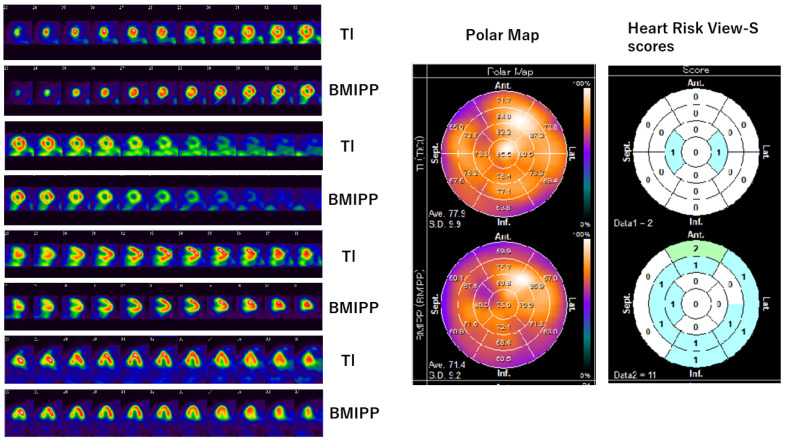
Tl/BMIPP dual-isotope myocardial scintigraphy of a patient. (Left) SPECT images are arranged in order from top to bottom: left ventricular short-axis planes of ^201^Tl and ^123^I-BMIPP, long-axis planes of ^201^Tl and ^123^I-BMIPP, and horizontal planes of ^201^Tl and ^123^I-BMIPP. (Middle) Polar map of ^201^Tl (top) and ^123^I-BMIPP (bottom). (Right) Heart Risk View-S scores of ^201^Tl (top) and ^123^I-BMIPP (bottom). Patient E in Table 2. Original image data can be found in Supplementary Materials (Figures S3 and S4).
